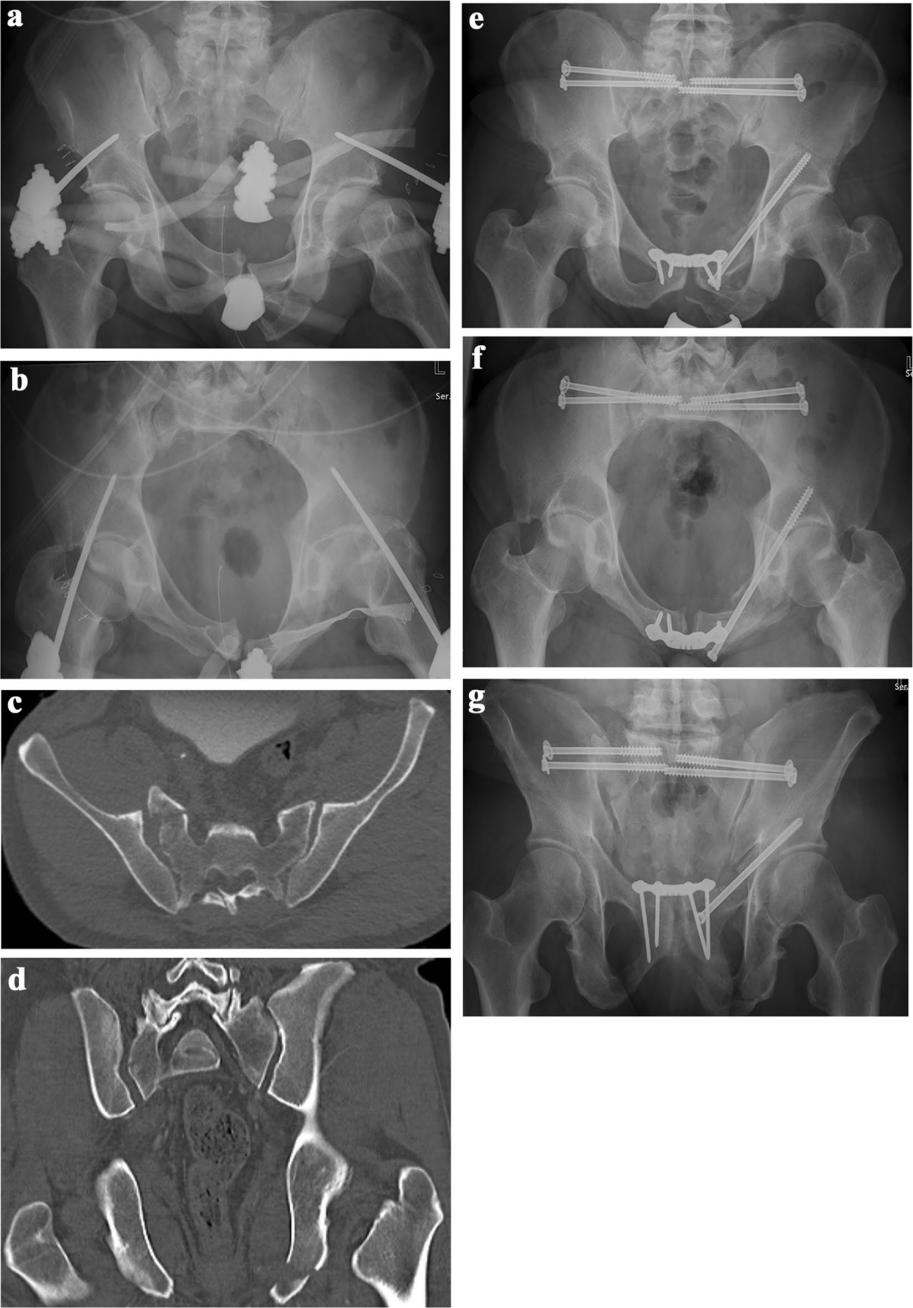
a 50-year-old male suffered an unstable pelvic ring injury due a traffic accident with high velocity. AP-view of the pelvis after application of an external fixator. A widening of the right iliosacral joint, a dislocation at the pubic symphysis and left-sided fractures of the superior and inferior pubic rami are visible. b Pelvic inlet view. A fracture at the anterior cortex of the right sacral ala and the displacement of the pubic symphysis are visible. c Axial CT-slice through the posterior pelvis. There is a widening of both iliosacral joints and a complete fracture through the right sacral ala. d Coronal CT reconstruction showing the widening of both iliosacral joints and left-sided fracture of the inferior pubic ramus fracture. e Postoperative AP-pelvic overview. Iliosacral joint disruptions and sacral fracture were stabilized with two iliosacral screws on both sides. Dislocation of the pubic symphysis was reduced and stabilized with plate and screw osteosynthesis. Left-sided superior pubic ramus fracture was reduced with a retrograde transpubic screw. There were no postoperative problems. No postoperative CT scan was made. f Pelvic inlet view. g Pelvic outlet view
